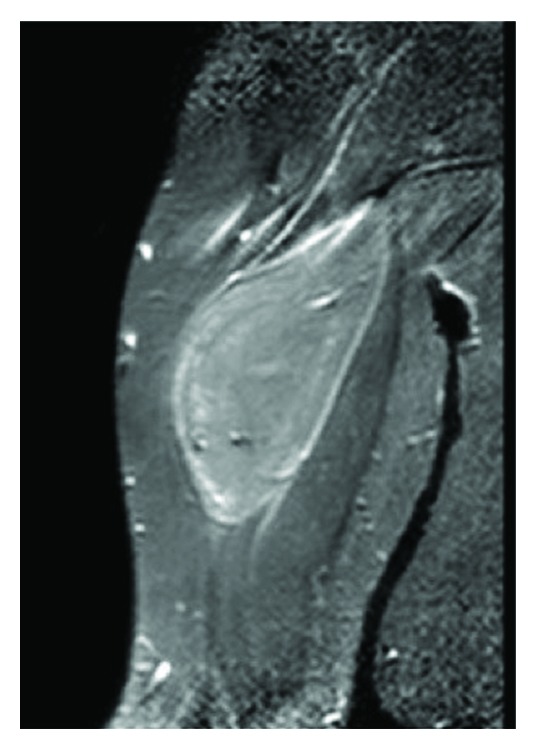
Coronal MRI image, contrast-enhanced, T2w.
